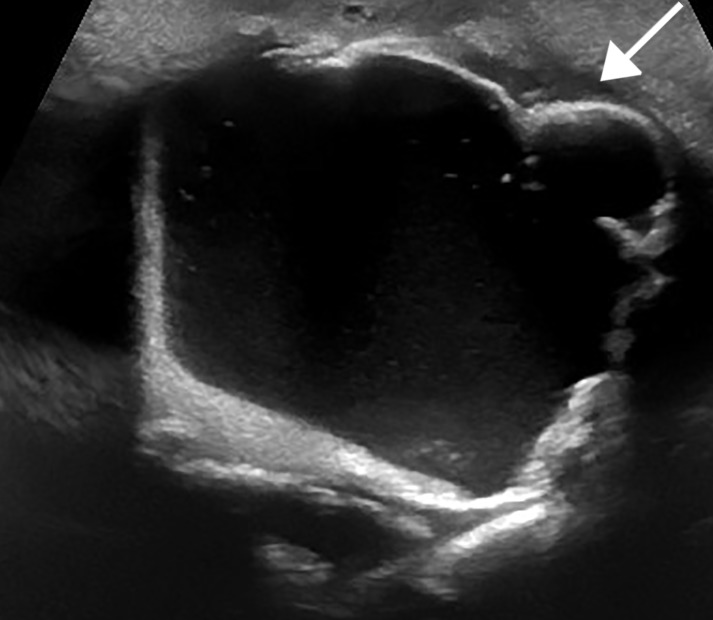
Right shoulder ultrasound Grayscale ultrasound image demonstrating large, echogenic, lobulated calcifications (arrow) in the lateral right shoulder.
